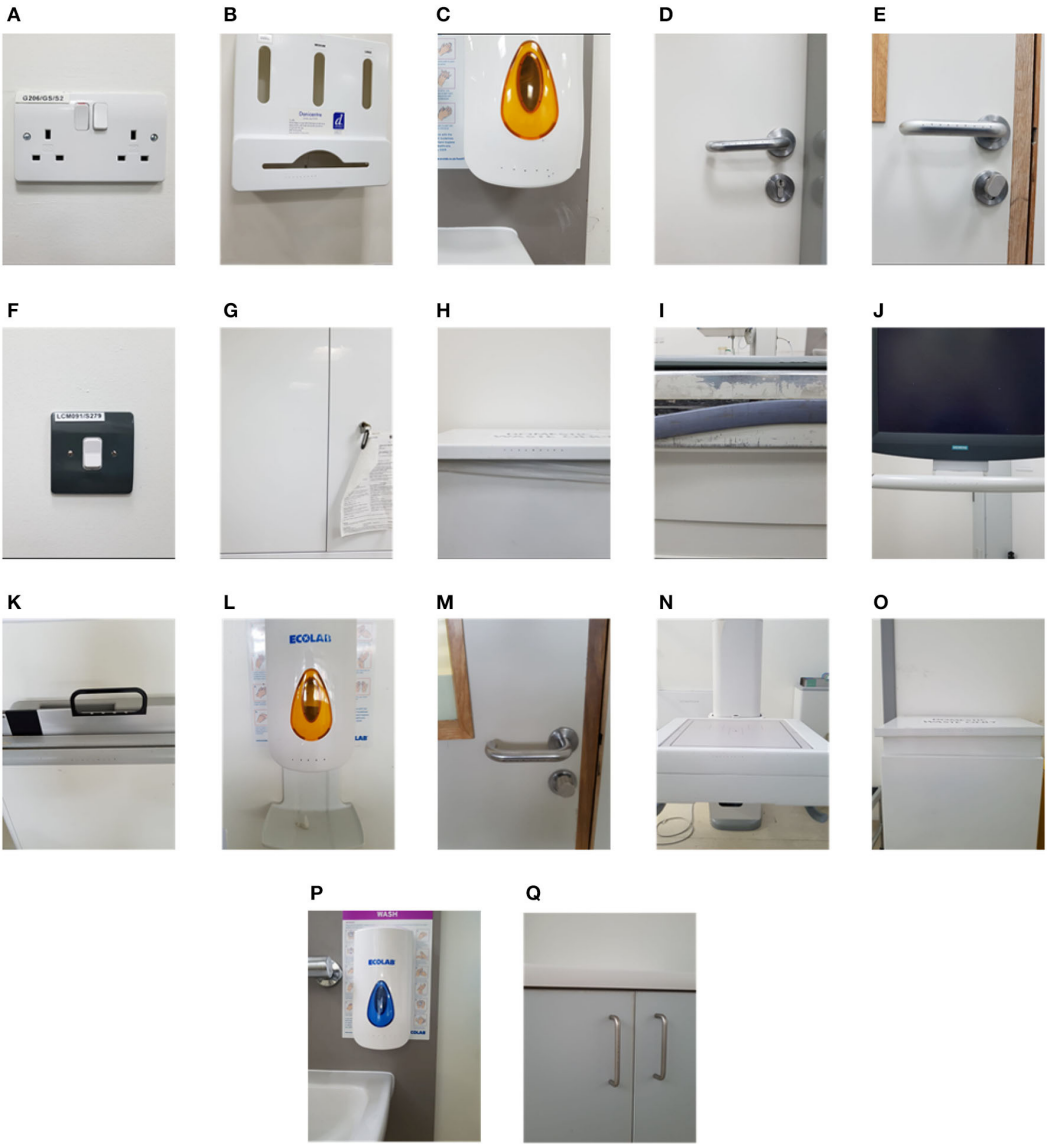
Commonly touched surfaces chosen for swab testing in hospital 1: (A–J) CT scan room; (K–Q) interventional suite.
